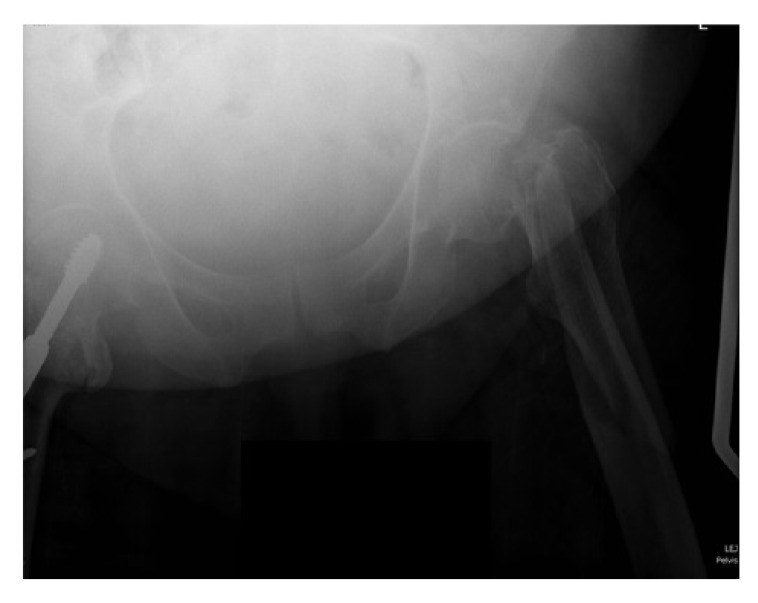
Anterio-posterior pelvic radiograph of Case 3 following the fall (a).
